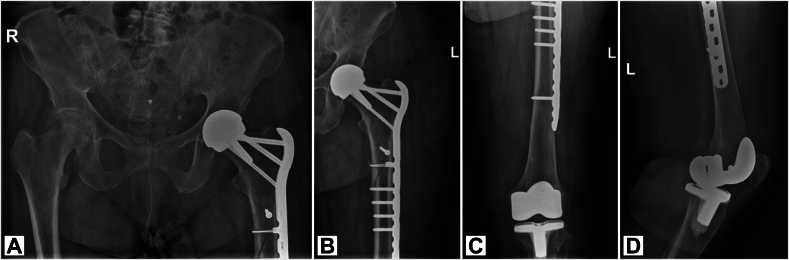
Postoperative radiographs at six weeks after surgery, showing the prosthesis and the Locking Compression Plate with cortical screws and cerclages. The femur is in anatomical position and shows signs of callus formation at the height of the fracture. A: Anterior-Posterior X-ray of Pelvis. B: Lauenstein X-ray of proximal femur. C: AP X-ray of distal femur. D: Lateral X-ray of left knee, showing a total knee arthroplasty.
